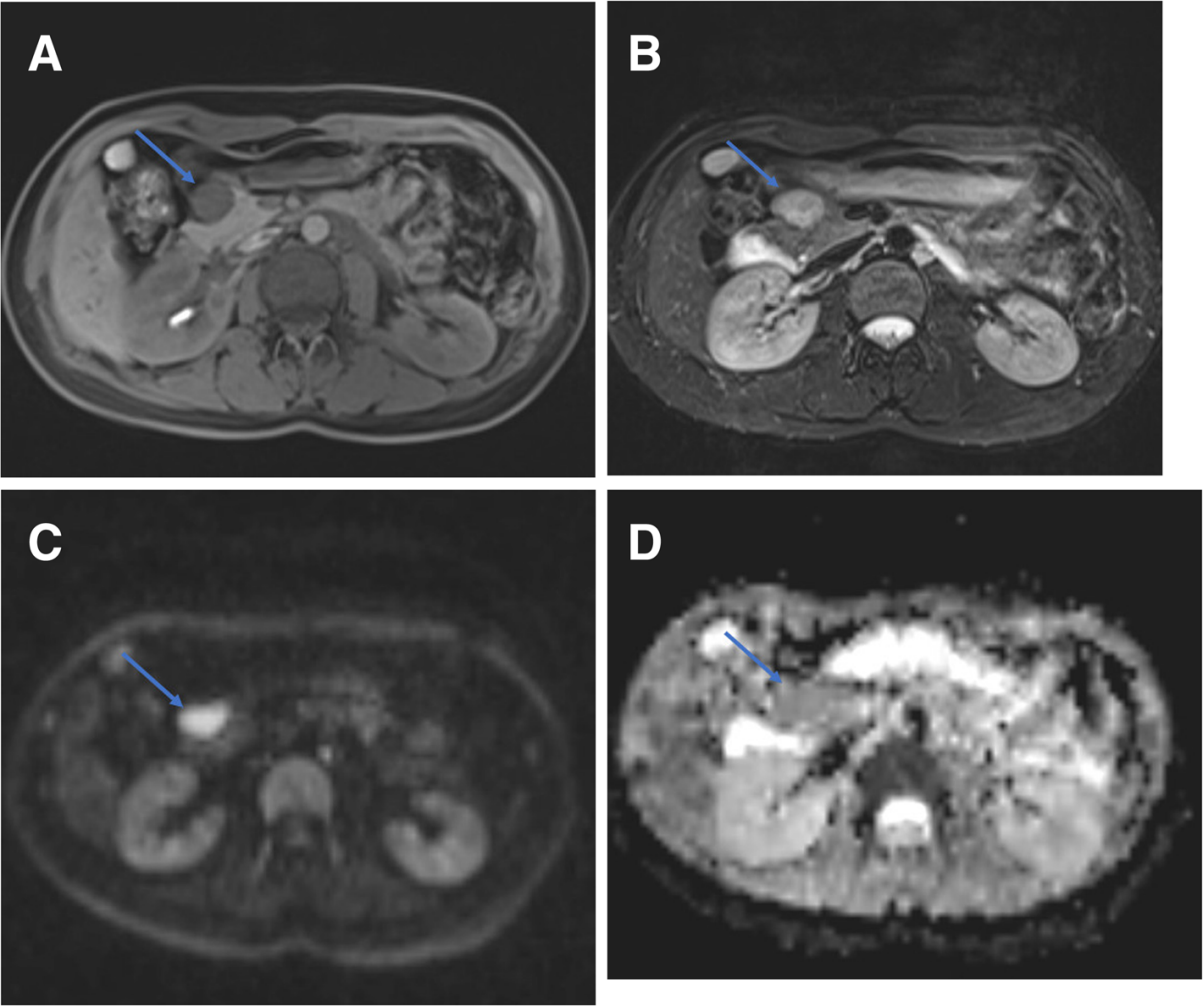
A well-circumscribed mass is seen in the head of the pancreas. The lesion is uniform hypo-intense on T1WI (a), homogeneous hyper-intense on T2WI (b), marked hyper-intense on DWI (b = 800 mm 2 /s) (c), and hypo-intense on ADC maps relative to the surrounding pancreas (d)
